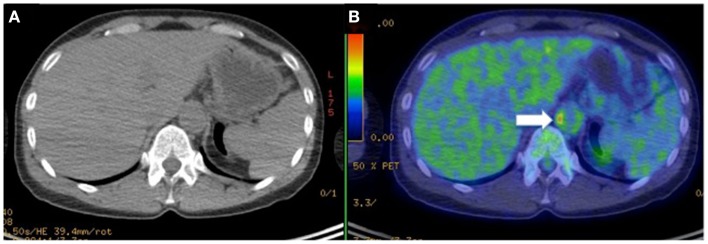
Hybrid CT (A) with PET imaging (B) to identifies [^18^F]-fluorodeoxyglucose (FDG) accumulation in the vascular wall of abdominal aorta (arrow) in a female patient with Takayasu arteritis.
